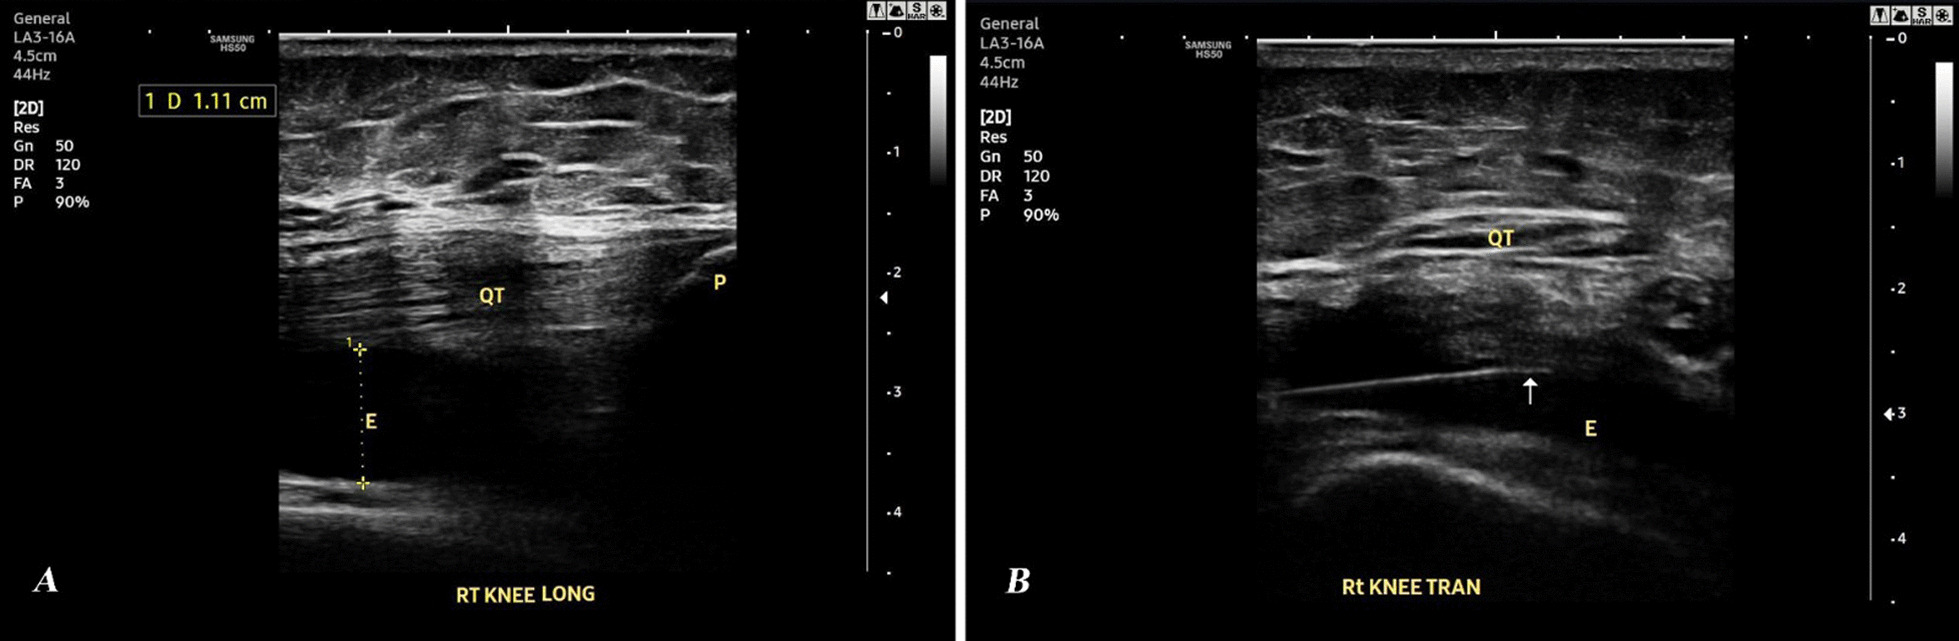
Ultrasound of the right knee of a 58-year-old knee osteoarthritis female patient showing effusion grade 3 and synovitis grade 2. A Longitudinal scanning. B Transverse scanning with 22-gauge spinal needle (white arrow). *QT* Quadriceps Tendon, *P* Patella, *E* Effusion
